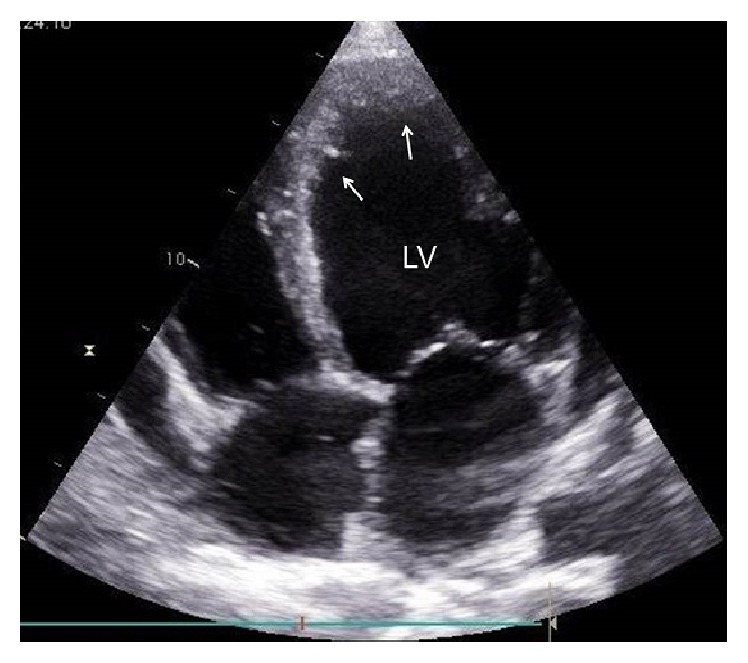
Echocardiographic apical 4-chamber view showing an enlarged left ventricle (LV) with hypertrabeculation/noncompaction affecting the left ventricular apex, the apical septum, and lateral wall (arrows).
